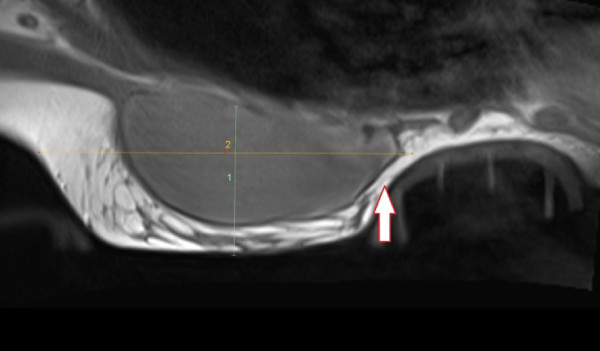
Sagittal MRI view mid-breast of an E-cup primary augmented individual in a size-1 device. The arrow points to the implant loading upon the orthosis, as it is too small. This must be avoided.
